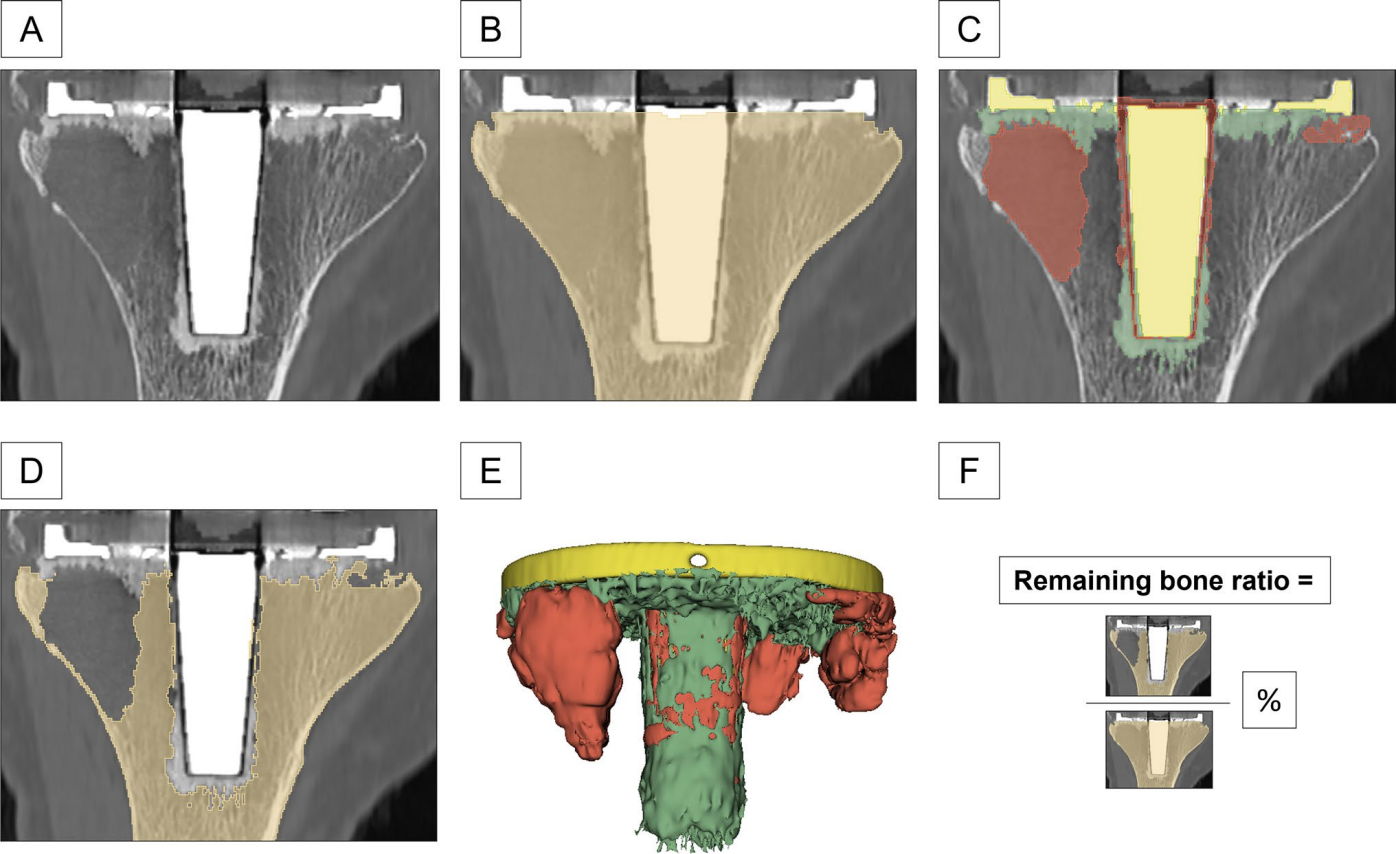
Three-dimensional defect segmentation. A Preoperative 3D-CT scan of a big epi- and metaphyseal defect in anterior–posterior view. B The total tibial bone (CT volume between the most distal part of the depicted tibia and the tibial cut) was defined as region of interest (ROI). C The volume of that ROI was separated in bone (light brown), implant (yellow), cement (green) and defect (red). E The total defect volume (cm^3^) was calculated by adding the volumes of the segmented implant, the cement, and the defect. D The volume of the remaining tibial bone after removal of the implant was obtained by subtracting the total defect from the tibial bone. Each segmentation was performed in three dimensions. Remaining bone ratio was calculated by dividing the ratio of the remaining tibial bone volume and the total tibial bone volume (F)
